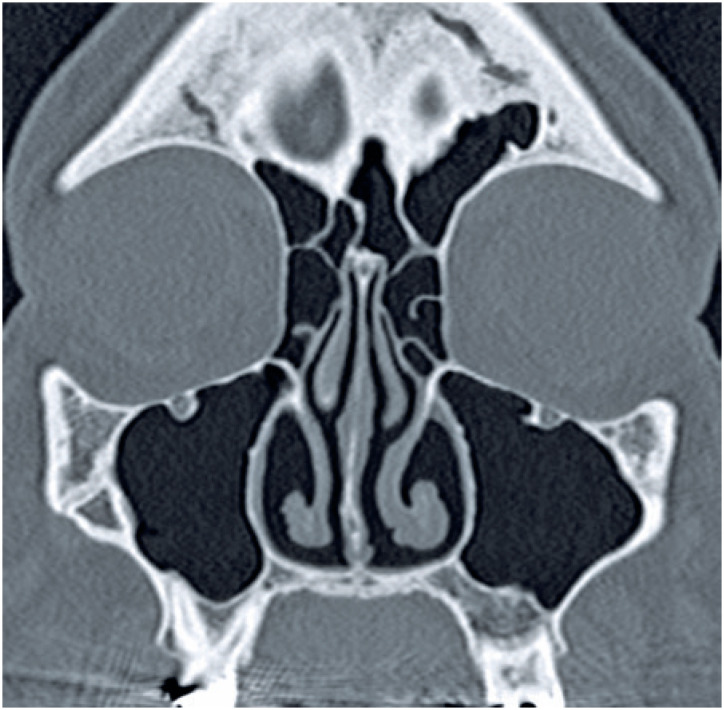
Coronal non-enhanced computed tomography scan of a severe acute respiratory syndrome coronavirus 2 positive patient shows normal aeration of the paranasal sinuses (score 0)
